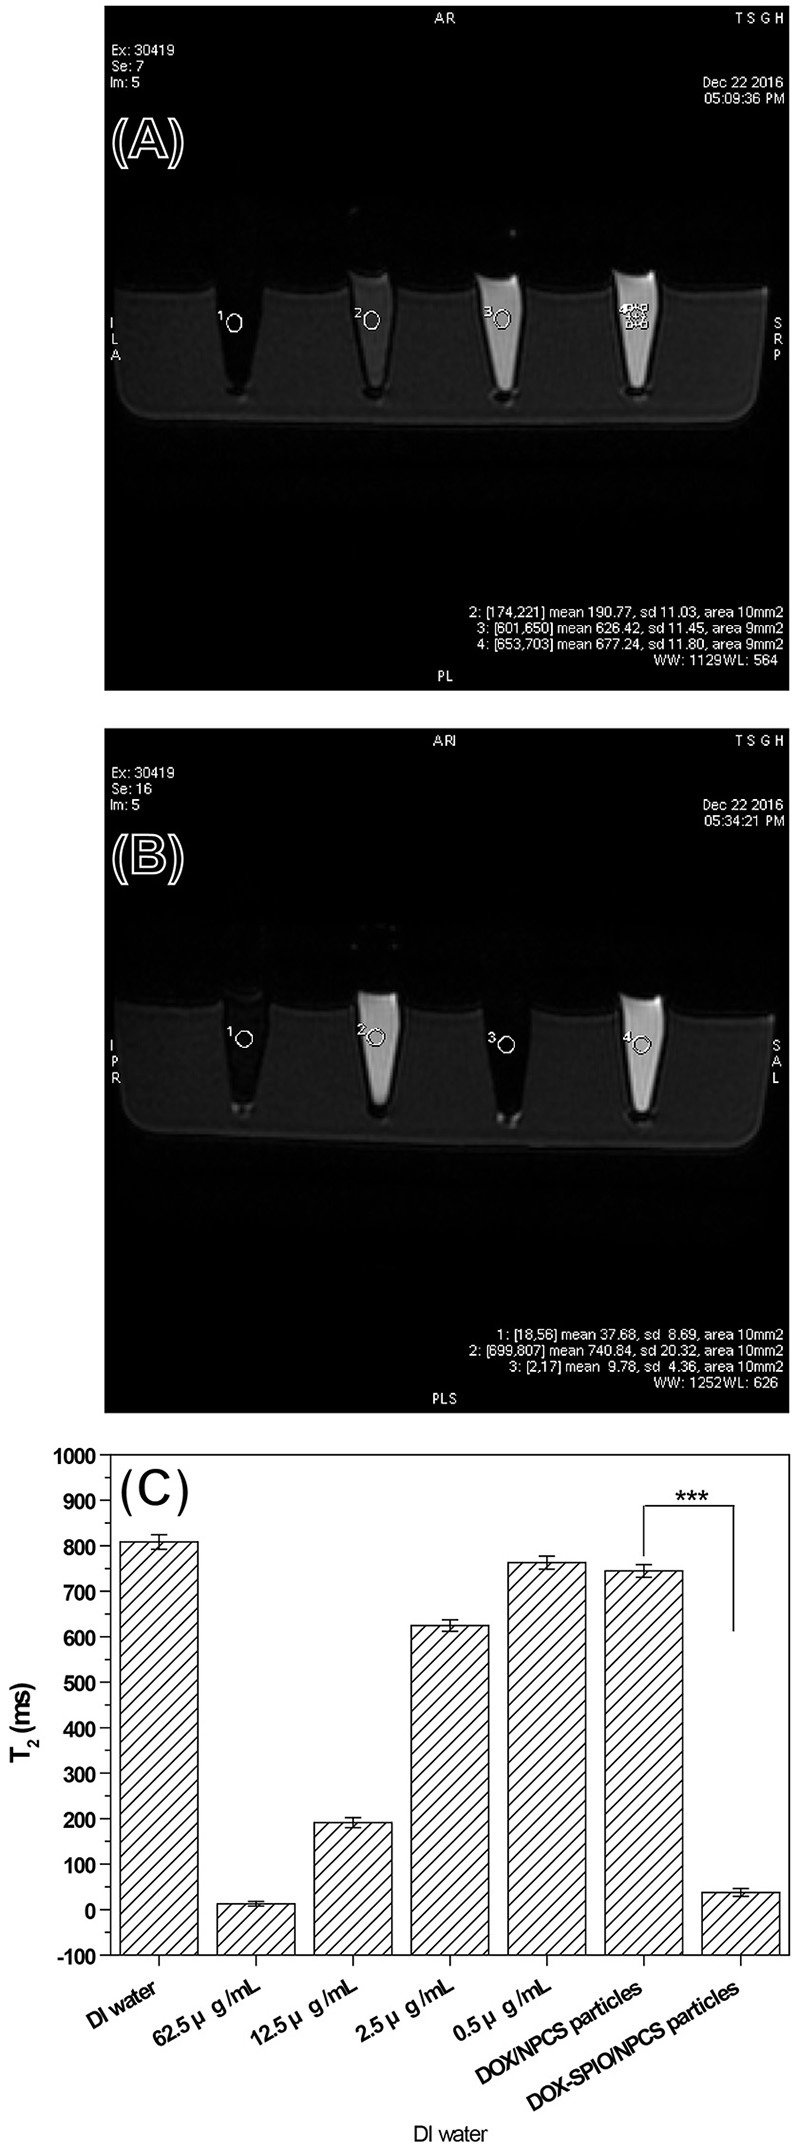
Signals of T2-weighted FSE MRI images from a series samples: (A) a series of DOX solution with concentration ranging from 0.5 to 62.5 μg/mL, (B) a series of DOX-SPIO/NPCS microparticle suspension with concentration ranging from 0 to 0.5 μg/mL and (C) quantatative value of T2 relaxation time.
